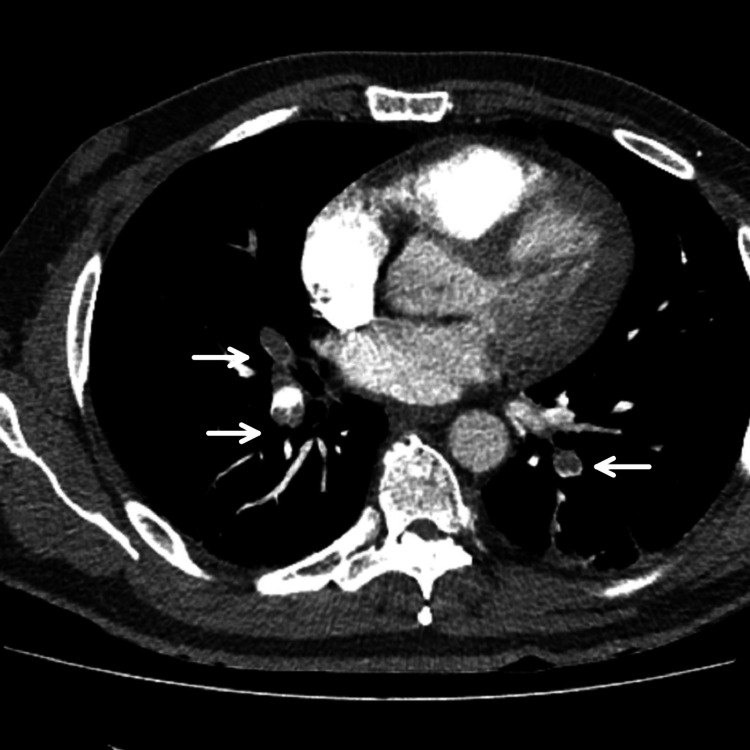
Computed tomography pulmonary angiography (CTPA) documented massive pulmonary embolism with intraluminal filling defects (arrows) of the right and left pulmonary arteries
